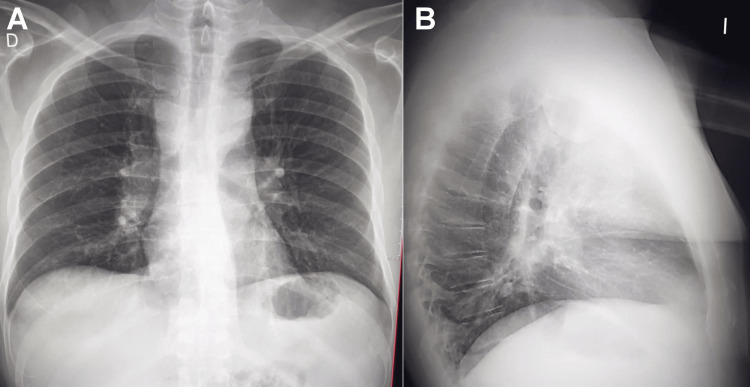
Posteroanterior and lateral chest radiographs demonstrating (A) mediastinal widening with prominence of the aortopulmonary window; (B) findings consistent with hilar lymphadenopathy.
